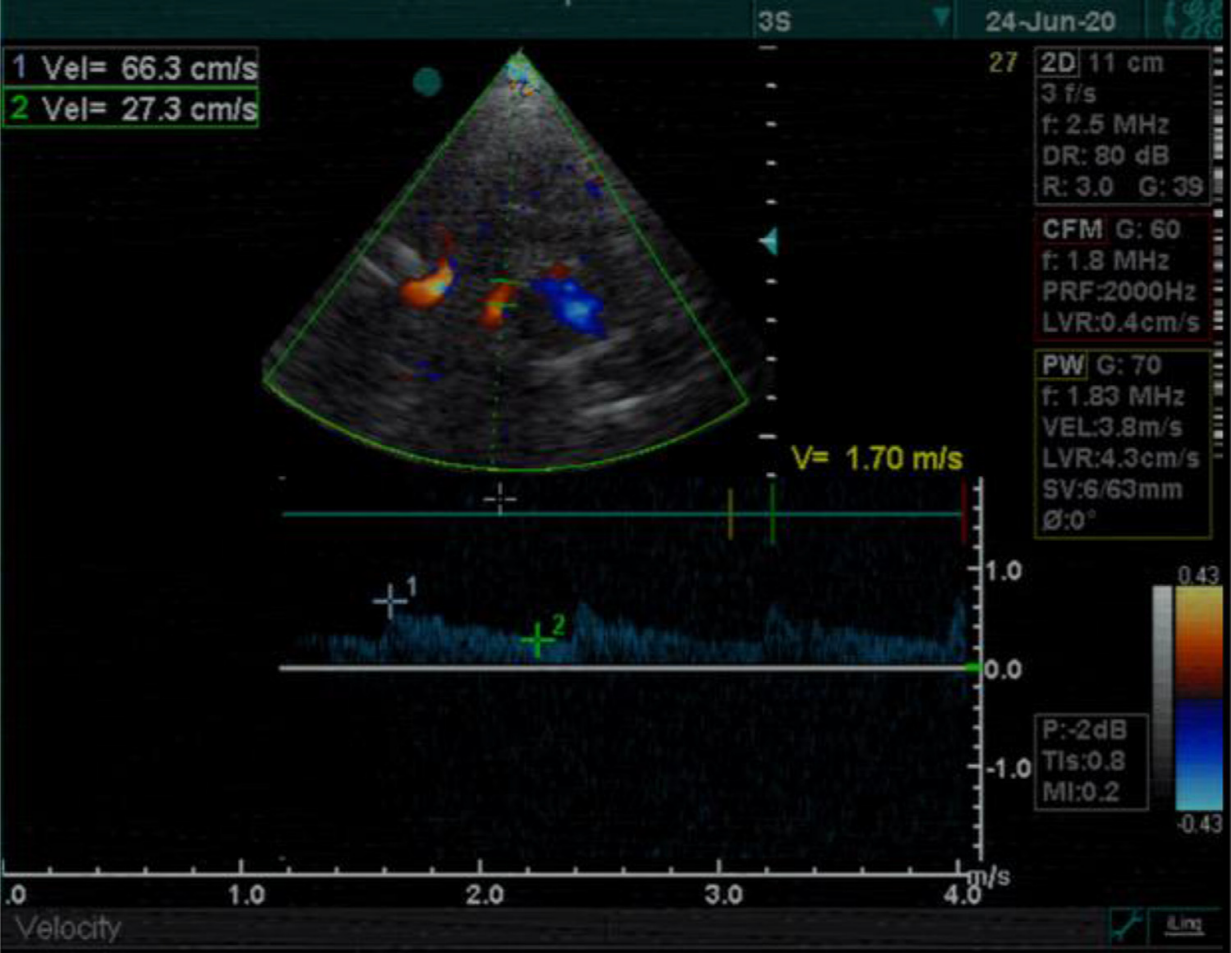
Intracranial Doppler ultrasonographic examination at the level of the left P1-P2 segment shows an attenuated flow pattern compared to the contralateral side (not shown).
